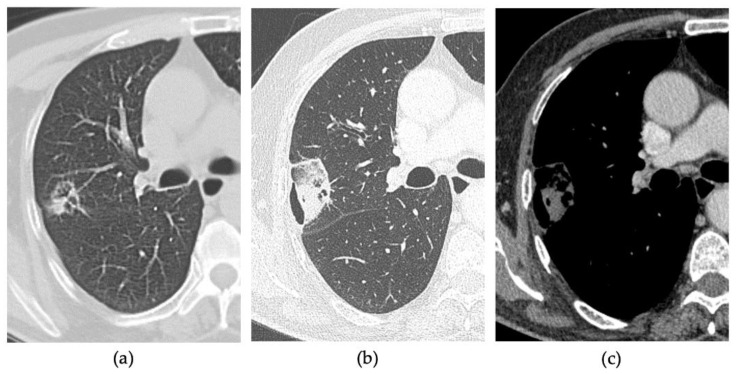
80-years-old man with lung metastasis from larynx carcinoma in the right upper lobe. (a) Axial pre-treatment CT. Axial 1-month follow-up CECT after MWA with VPS shows (b) a large consolidation with well-defined margins and cavitation and (c) with contrast enhancement.
